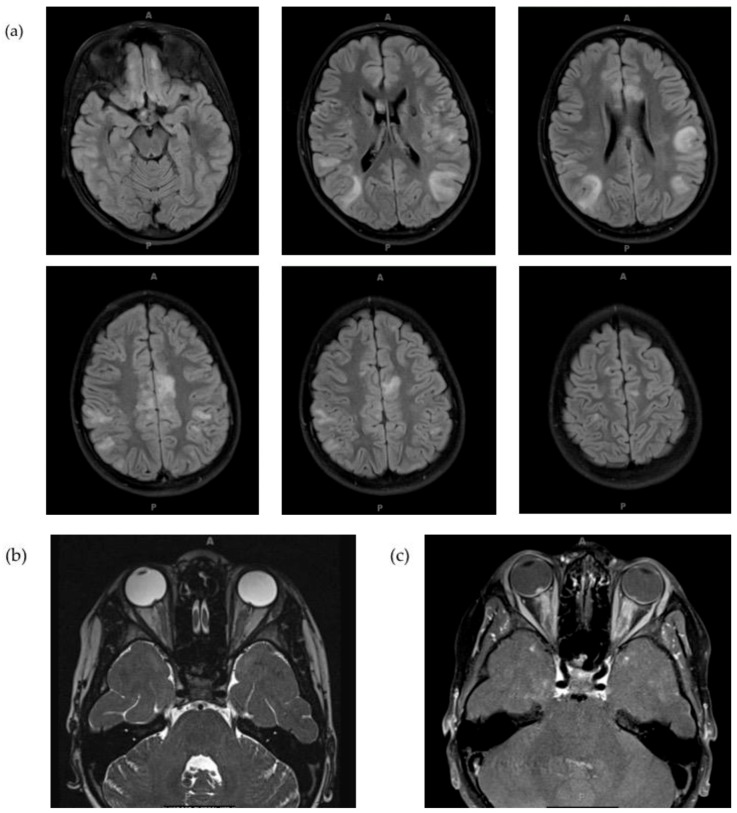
Brain MRI of patient at initial demyelinating event. (a) Multiple areas of large diffuse cortical and juxtacortical T2/FLAIR hyperintensities involving bilateral frontal, temporal, parietal and occipital areas. (b)Thickening of the optic nerves with abnormal T2 signal of the bilateral intraorbital, canalicular and prechiasmatic optic nerves with evidence of bulging of the optic disc and flattening of the posterior sclera. (c) Contrast enhancement.
